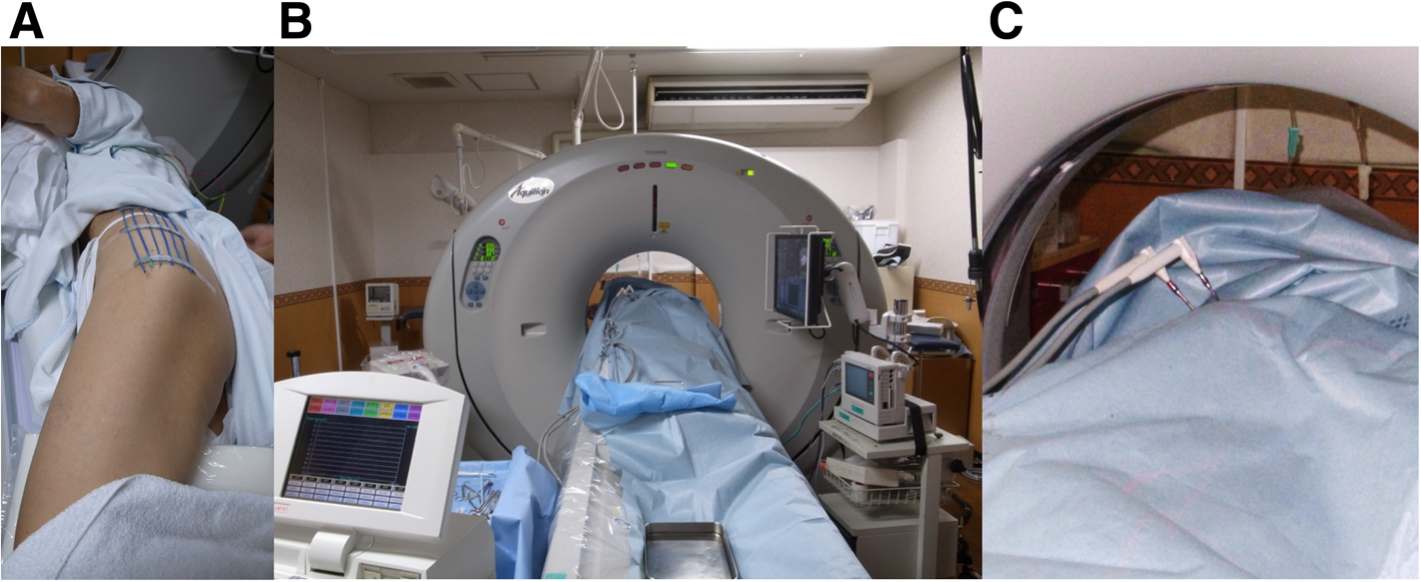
a, b Markers were placed on the left buttock to discern the optimal direction of probe insertion and cryoablation was performed under CT guidance. c Two probes were inserted into the lesion, and an ice ball was monitored to secure at least a 1-cm margin around the tumor
